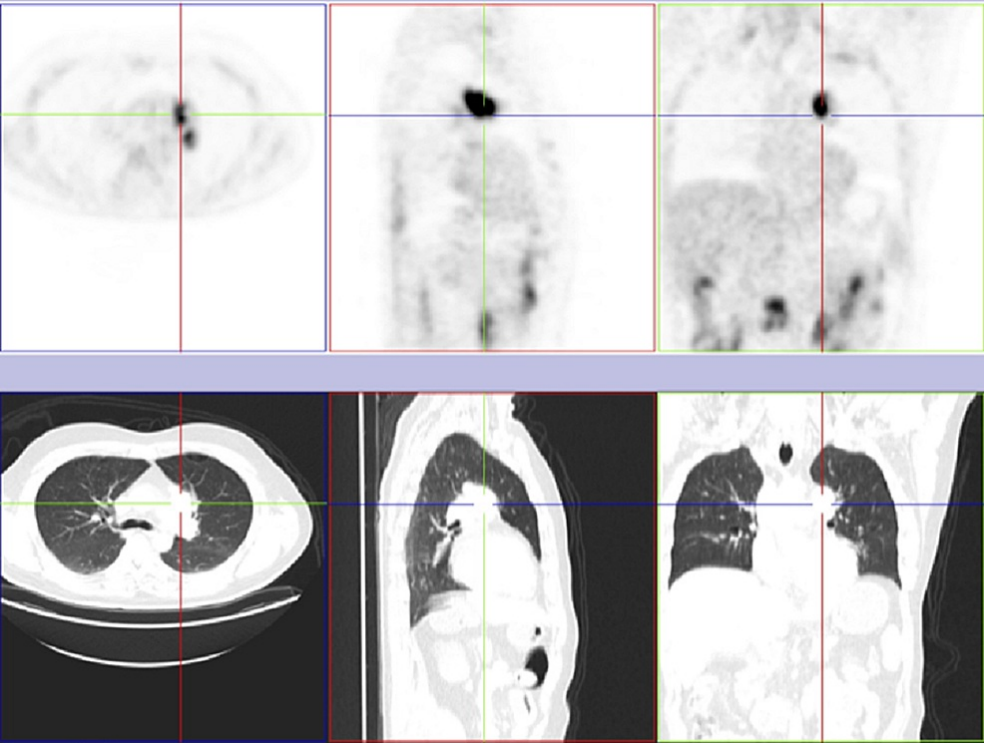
18F-FDG PET and CT scan showing the pulmonary lesion and aortopulmonary lymphadenopathies. 18F-FDG PET: 18F-fluorodeoxyglucose positron emission tomography
